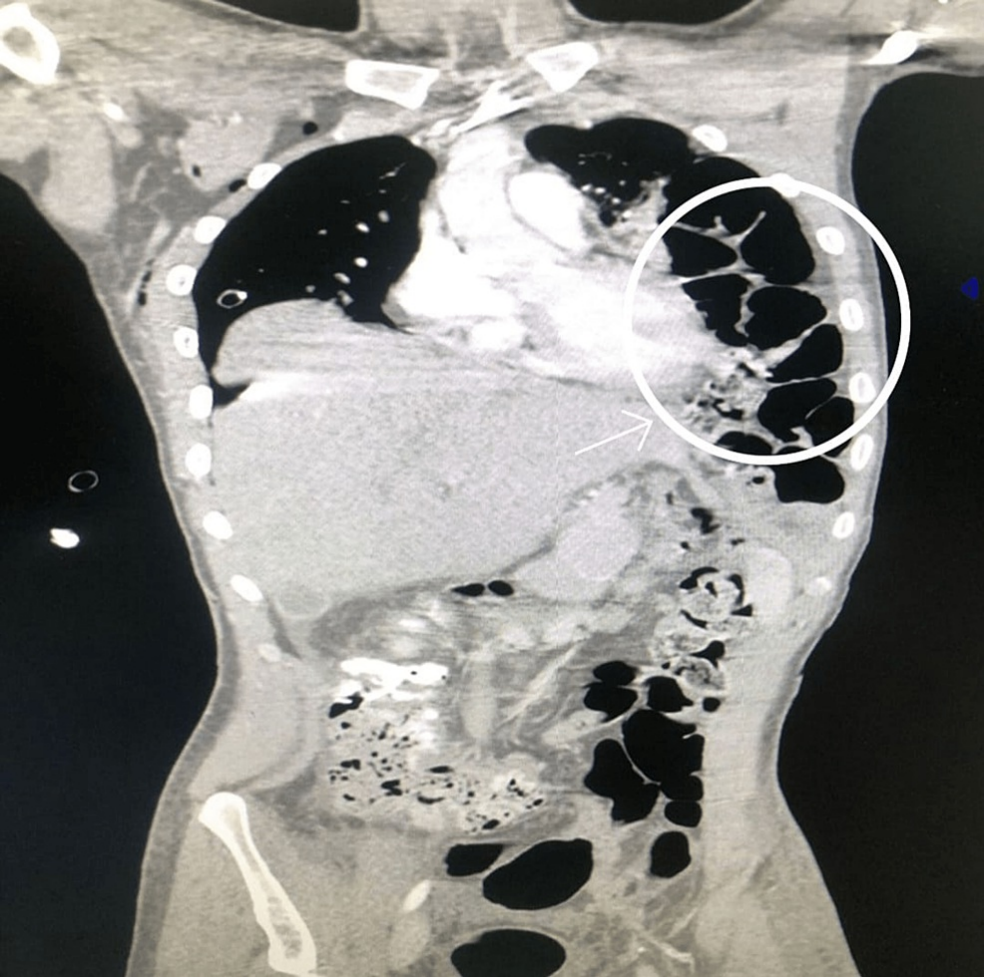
Computed tomography of the chest showing herniation of bowels in the left hemithorax.
